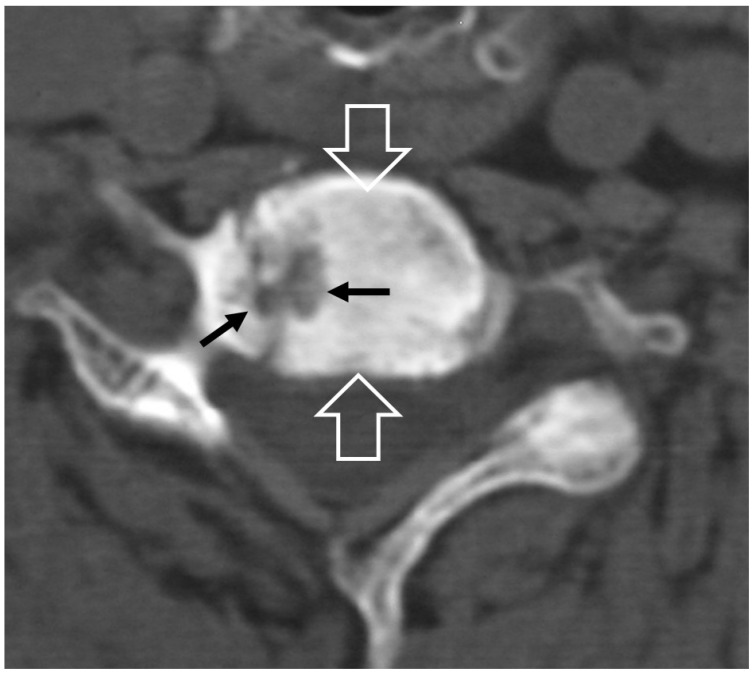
A 65-year-old chronic renal failure female patient, undergoing hemodialysis for 12 years. She has been complaining of neck pain for 6 months. Axial CT showing osteolytic lesions (arrows) and osteosclerosis (open arrows) at the C6 vertebra.
